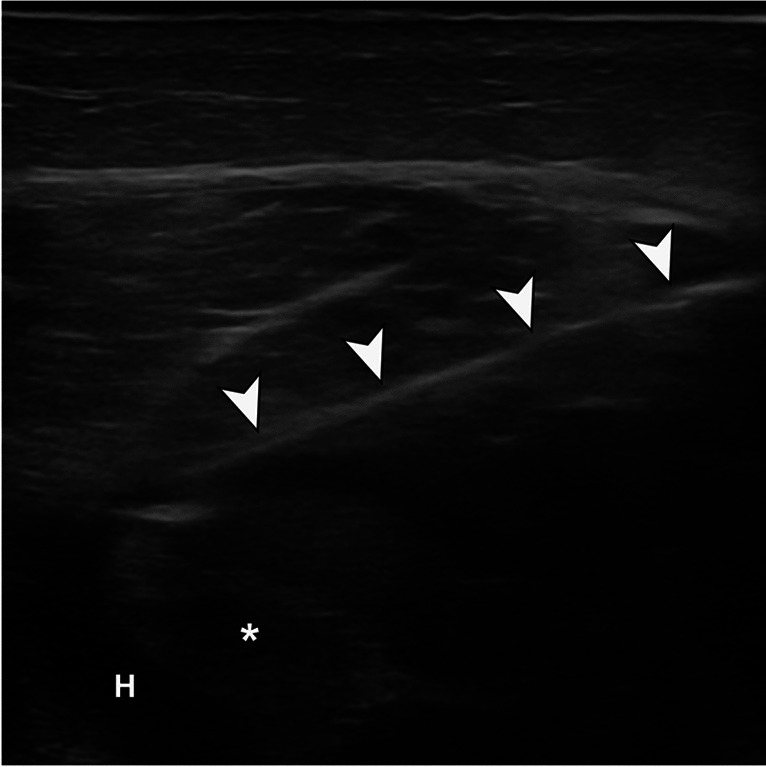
Ultrasound-guided aspiration of the hematoma with an in-plane transversal and posteromedial approach. Needle (arrowheads) has the distal tip at the hematoma (H). Approximately 80 ml of hematic fluid was aspirated from the injury. Asterisk: degloved inner muscle fibers
